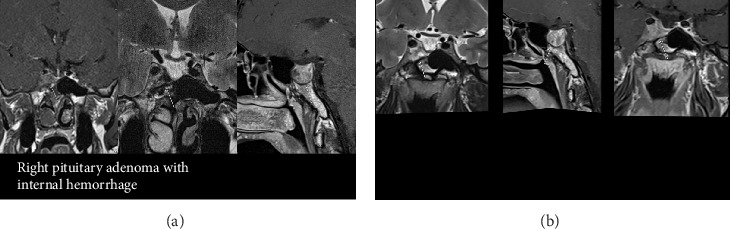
(A) Dcember 2021 MRI showing a pituitary tumour dimensions of 23.8 mm × 18.9 mm ×18 mm. (B) MRI December 2023 showing greater brightness of the pituitary tumour and reduction in size 21.3 mm × 18.3 mm × 17 mm.
